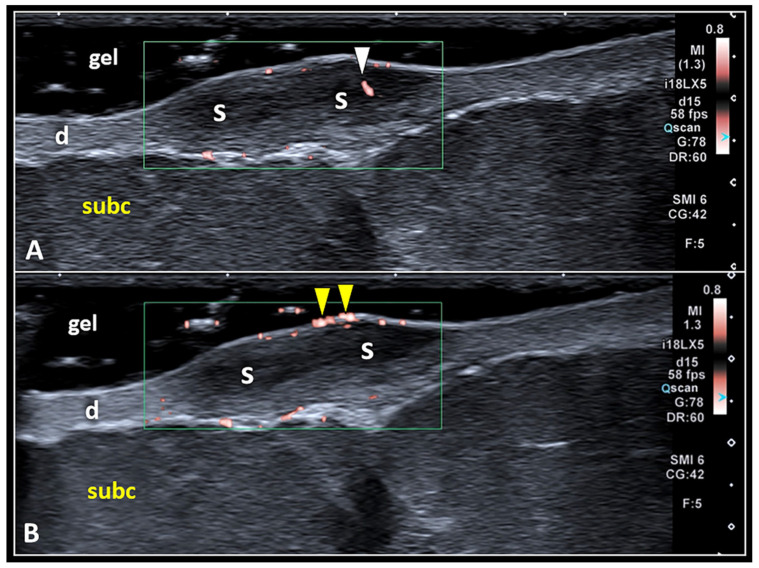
Pitfalls in Doppler imaging of SS. Using a large amount of gel (i.e., the suspension technique) and fully including the scarring tissue (S) inside the Doppler box, it is paramount to accurately differentiate the real vascular signals (white arrowhead) inside the scar (A) from the ‘fake’ ones (yellow arrowheads) generated by the acoustic interface between the epidermis and the gel (B). d: dermis, subc: subcutaneous tissue.
